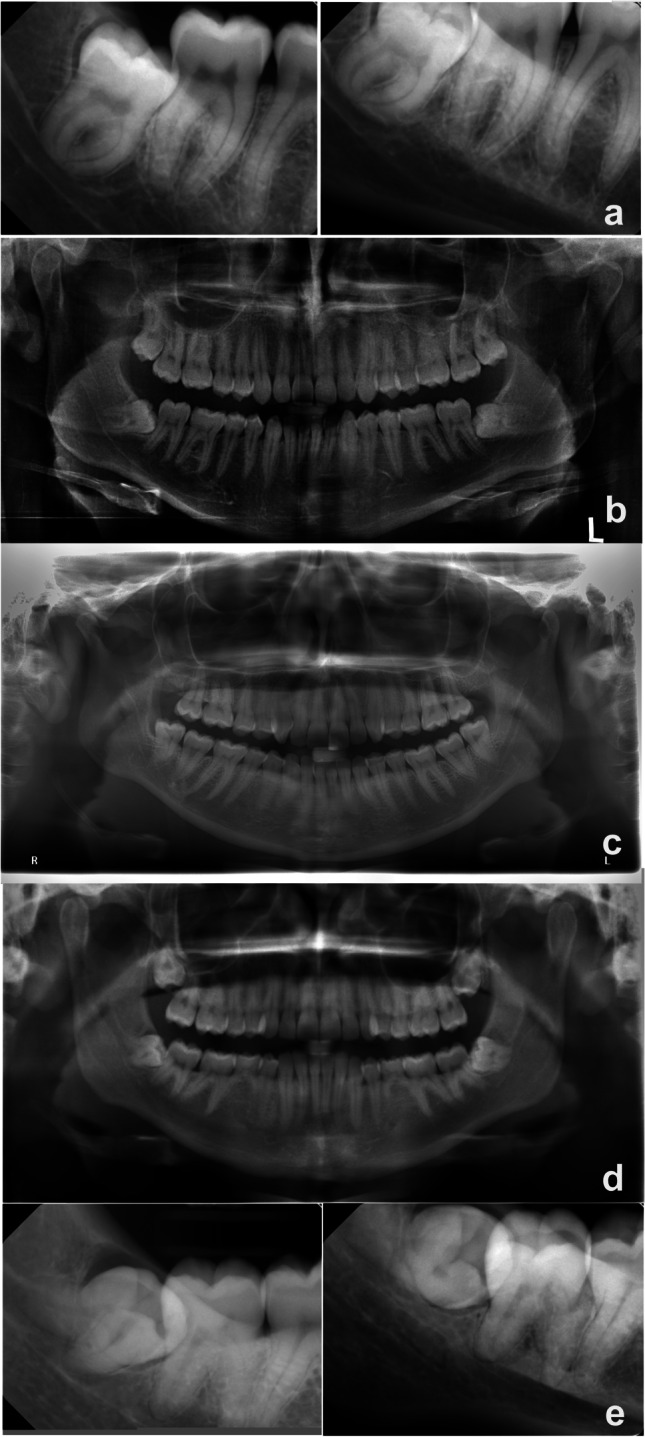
The radiographs presented in the survey. a Case I. b Case II. c Case III. d Panoramic radiograph for case IV. e Intraoral radiographs for case IV
